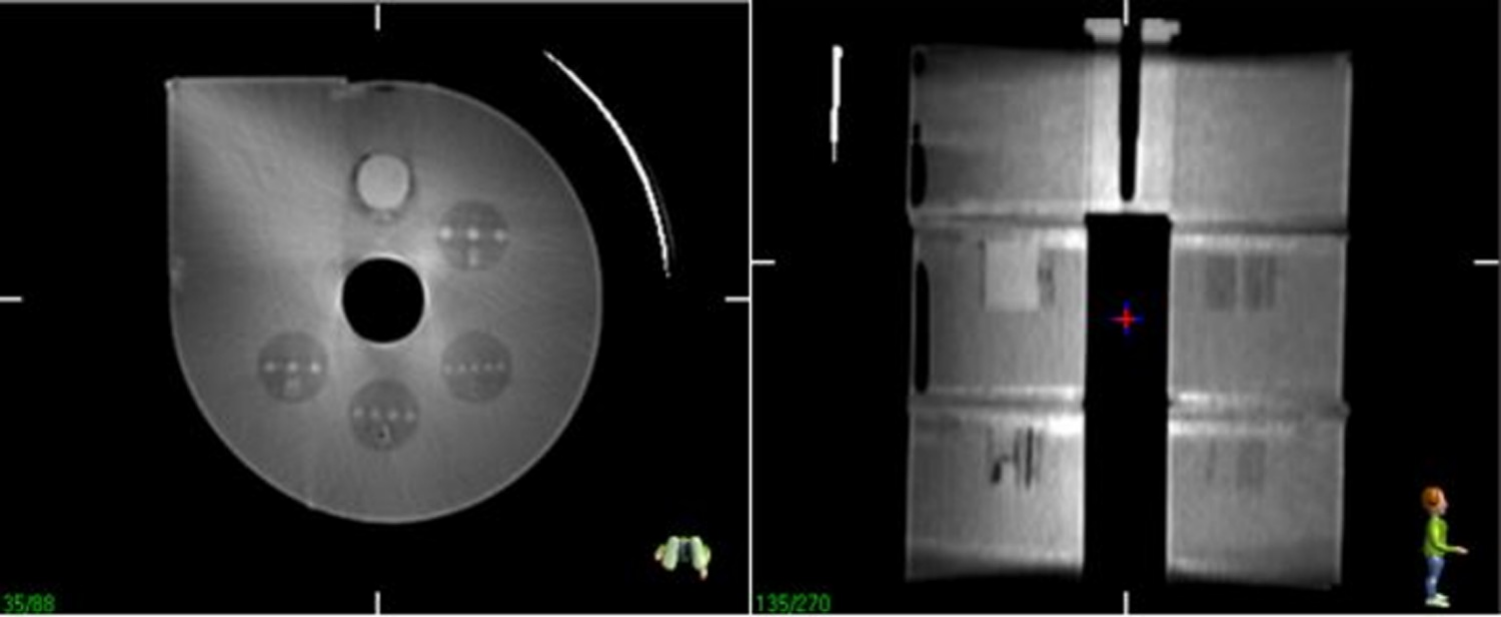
CBCT images of the low-contrast detectability slab.
